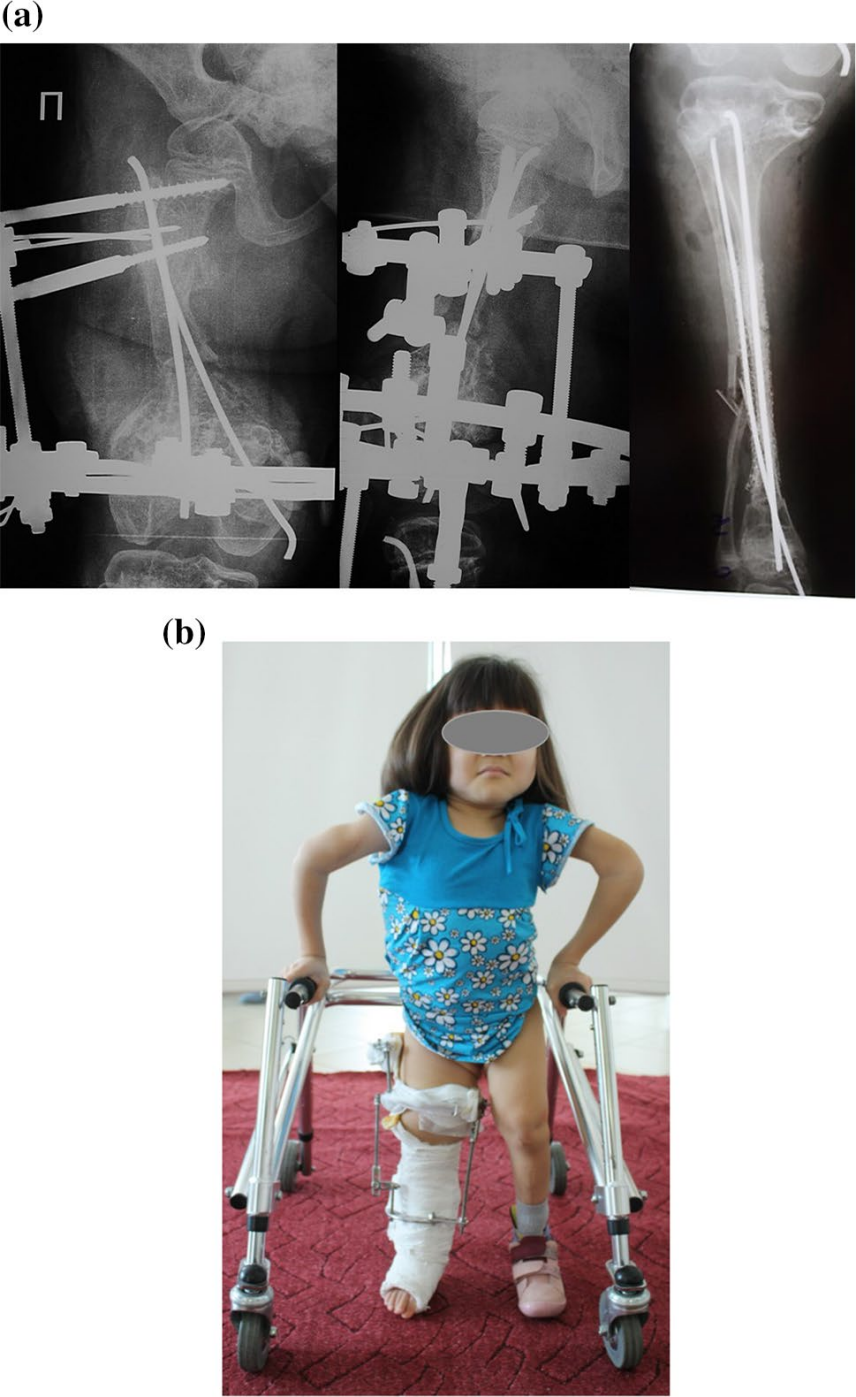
Surgery and postoperative period: a intraoperative radiographs of right femur and tibia; surgery consisted of Rush rod removal; FIN of femur associated with Ilizarov fixator and FIN of tibia associated with subperiosteal titanium nickelide mesh; plaster cast was applied at tibia and foot and integrated with Ilizarov fixator; b patient standing with walker in early postoperative period
